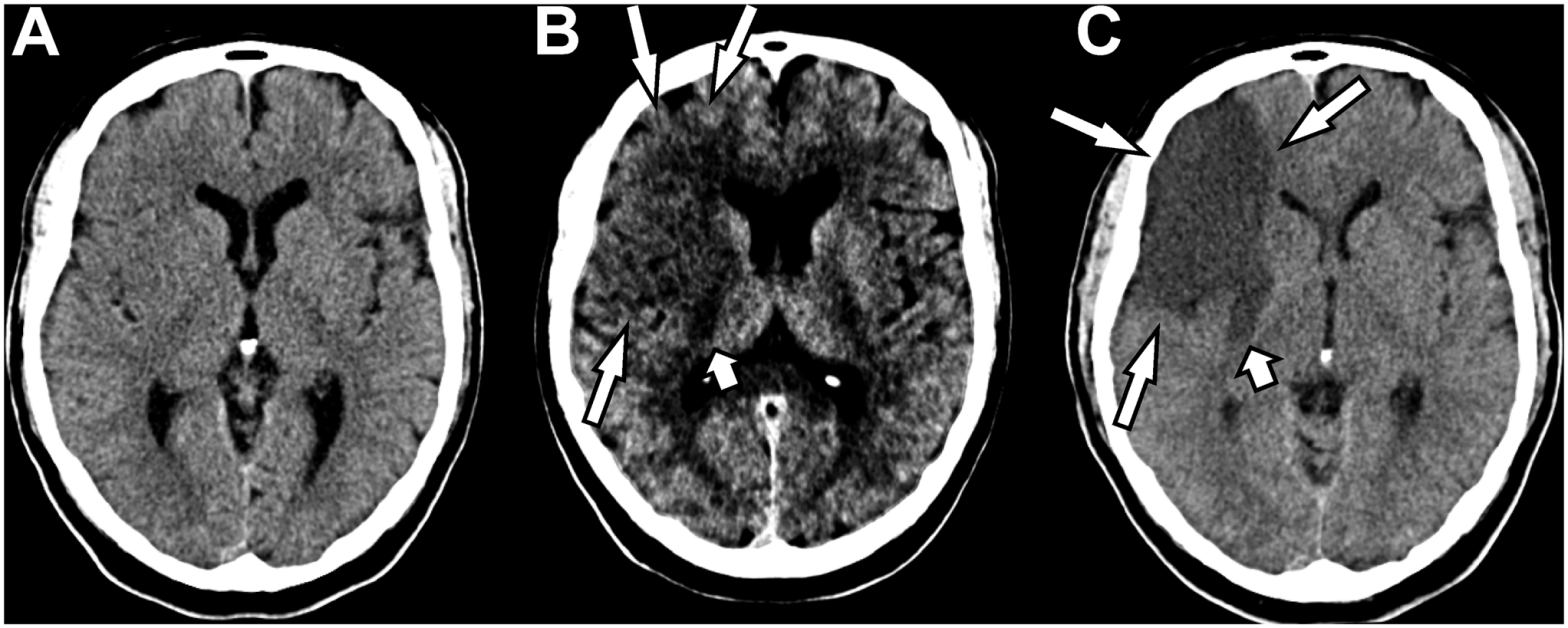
64 year-old male patient with ischemic stroke of the right cerebral hemisphere due to an isolated occlusion of the right internal carotid artery. While the NECT (A) only give an idea of a slight dedifferentiation of gray and white matter at the insular cortex, the “best contrast” image (B) shows a great delineated infarction (long arrows) with involvement of the internal capsule (short arrow). The diagnosis was confirmed on follow-up CT, which shows that the BC image reflects the infarct size more accurately (C).
